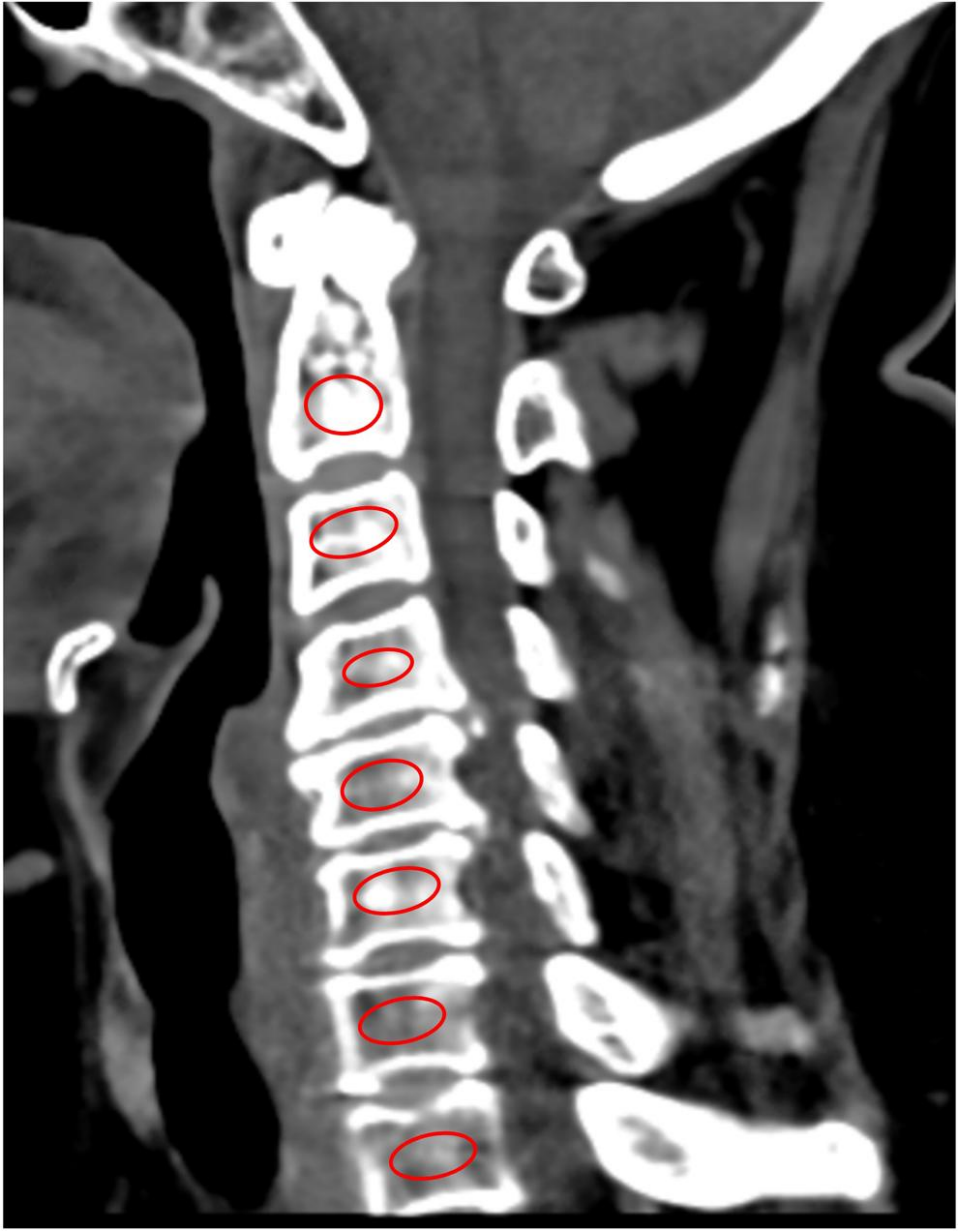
Sagittal computed tomography (CT) image of the cervical spine (C2–T1) showing the placement of elliptical regions of interest (ROIs) for hounsfield unit (HU) measurement. Each vertebral body from C2 through T1 is marked with a red ROI placed centrally within the trabecular bone. The ROIs are positioned to avoid inclusion of the surrounding cortical bone, endplates, and venous plexuses, ensuring that the measured HU values accurately represent trabecular bone density.
